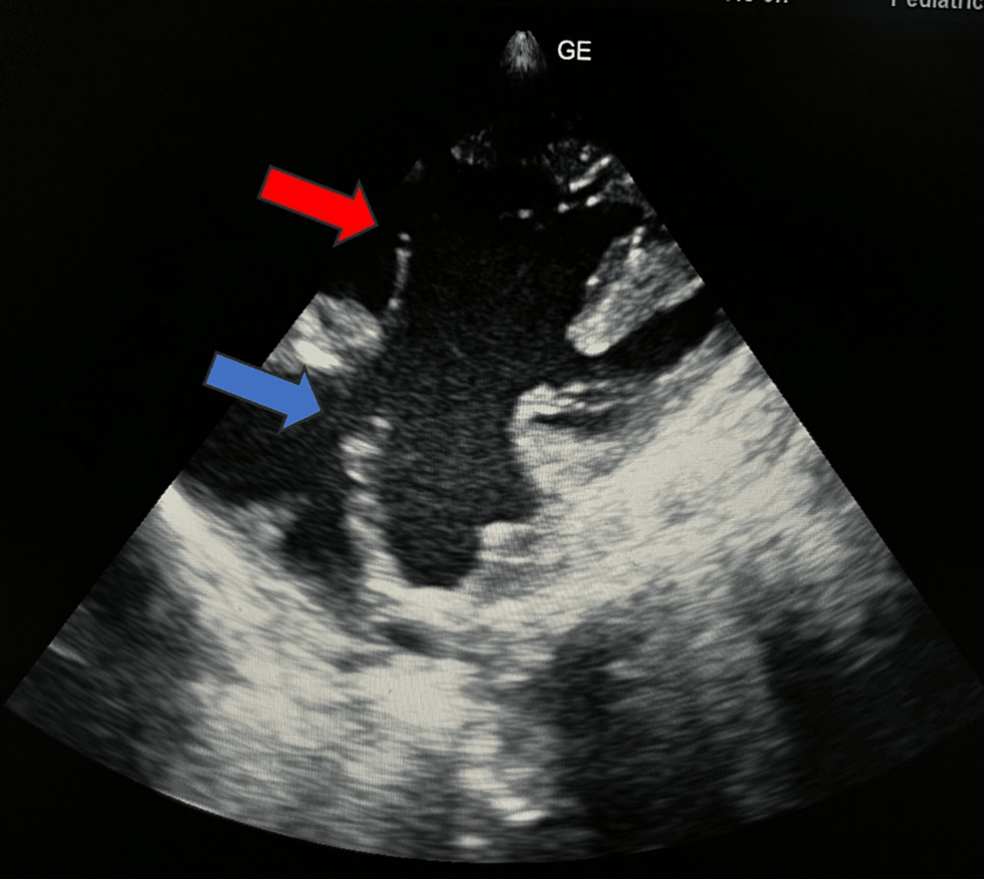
2D echocardiography apical four-chamber view shows large atrial septal defect (blue arrow) and large ventricular septal defect (red arrow).
